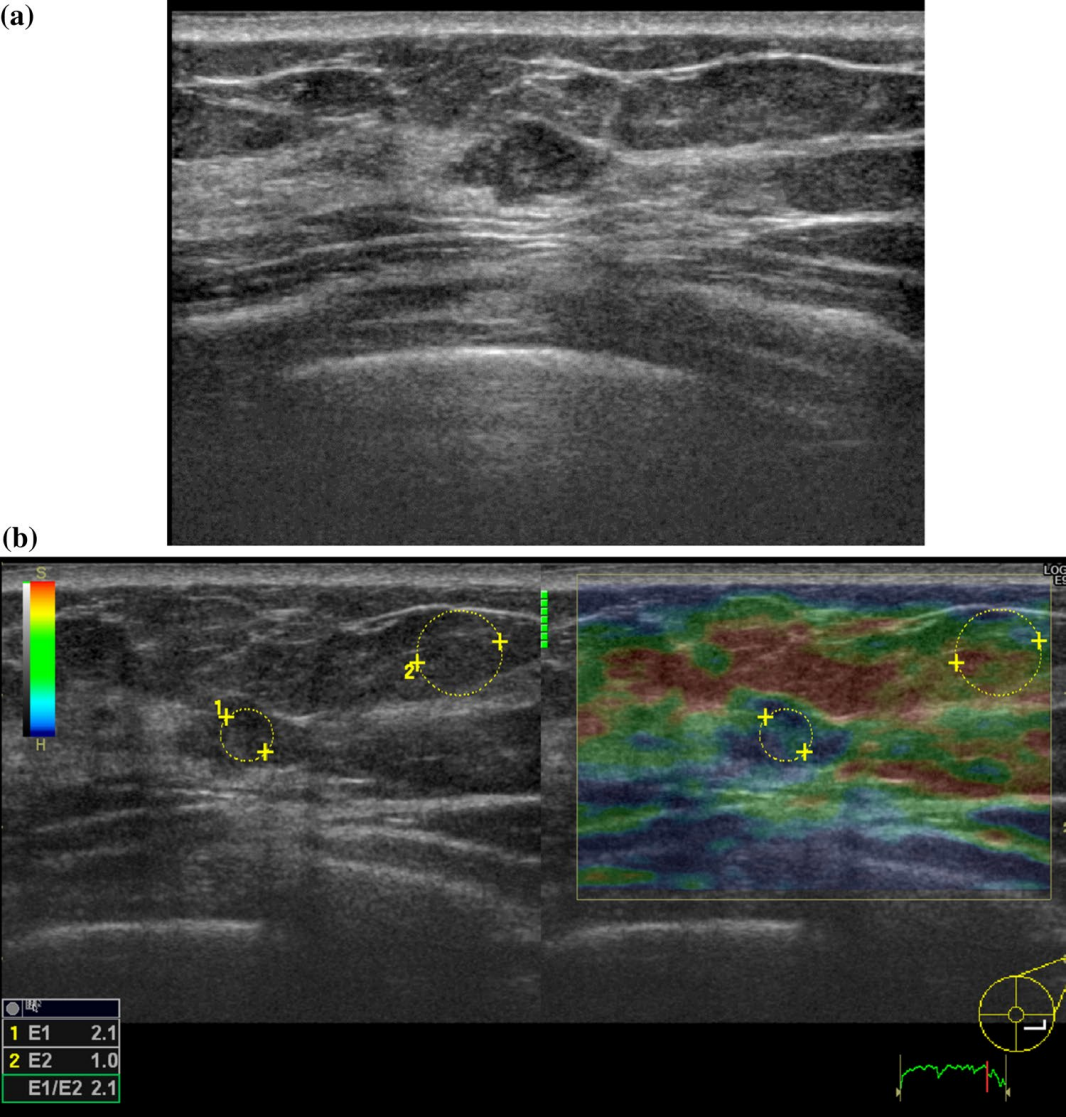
Ductal carcinoma in situ. a On B-mode ultrasound, there is a hypoechoic tumor that is relatively flat and slightly uneven inside. b The strain inside the tumor is uneven and has blue and green parts. E-index and E-ratio are both 2.1, which is much lower than the cut-off value
